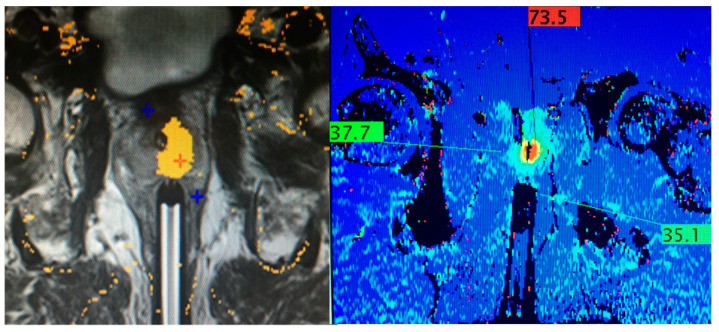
Thermal imaging during MRI-guided transrectal laser ablation of the left gland. (Left image): the yellow zone indicates irreversible tissue damage. Notice sparing of the central urethra due to cooling saline infusion through the indwelling Foley catheter. (Right image): real-time thermometry with electronic calipers indicating estimated temperatures during laser ablation. Above 60 °C, there is instant cell death. The lower right caliper monitors rectal wall temperature, shown as 35 °C (normal).
